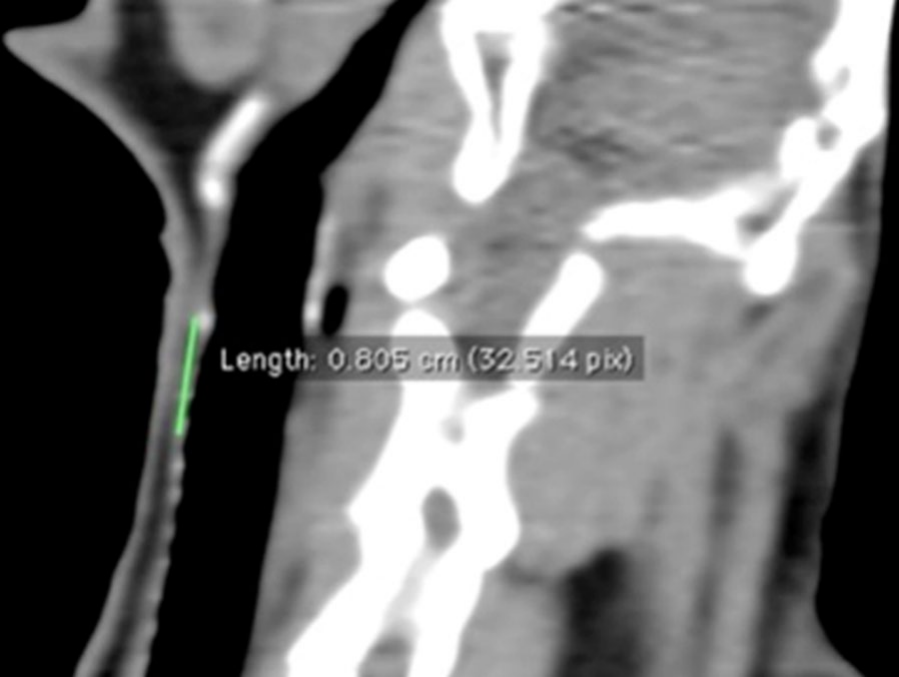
Sagittal CT image showing a distance of 8.1 mm from the cricoid cartilage to the third tracheal ring
